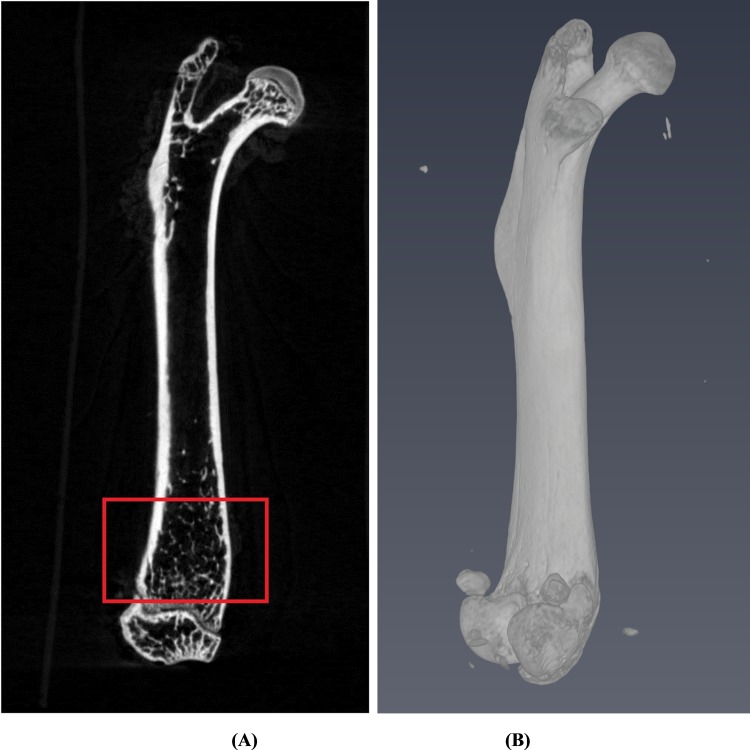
(A) The growth plate was first located at the distal femur site as a reference plane and delineated a 2 mm distance along the longitudinal direction as shown in the red box for our region of interest. (B) A 3-dimensional surface rendering figure of the whole femur bone which is reconstructed from micro CT images is shown.
